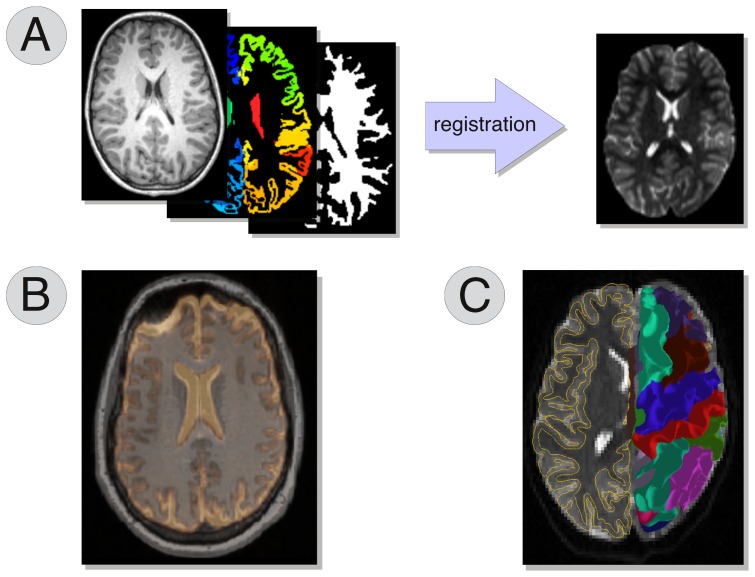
Registration between morphological and diffusion images. The reference space in CMP is the one of the diffusion images. The tissue masks extracted during the segmentation step, then, have to be registered to the diffusion space (A). The quality of the registrations can be inspected by overlaying on the b0 either the T1-weighted volume (B) or the geometric models of the cortex estimated with Freesurfer (C).
